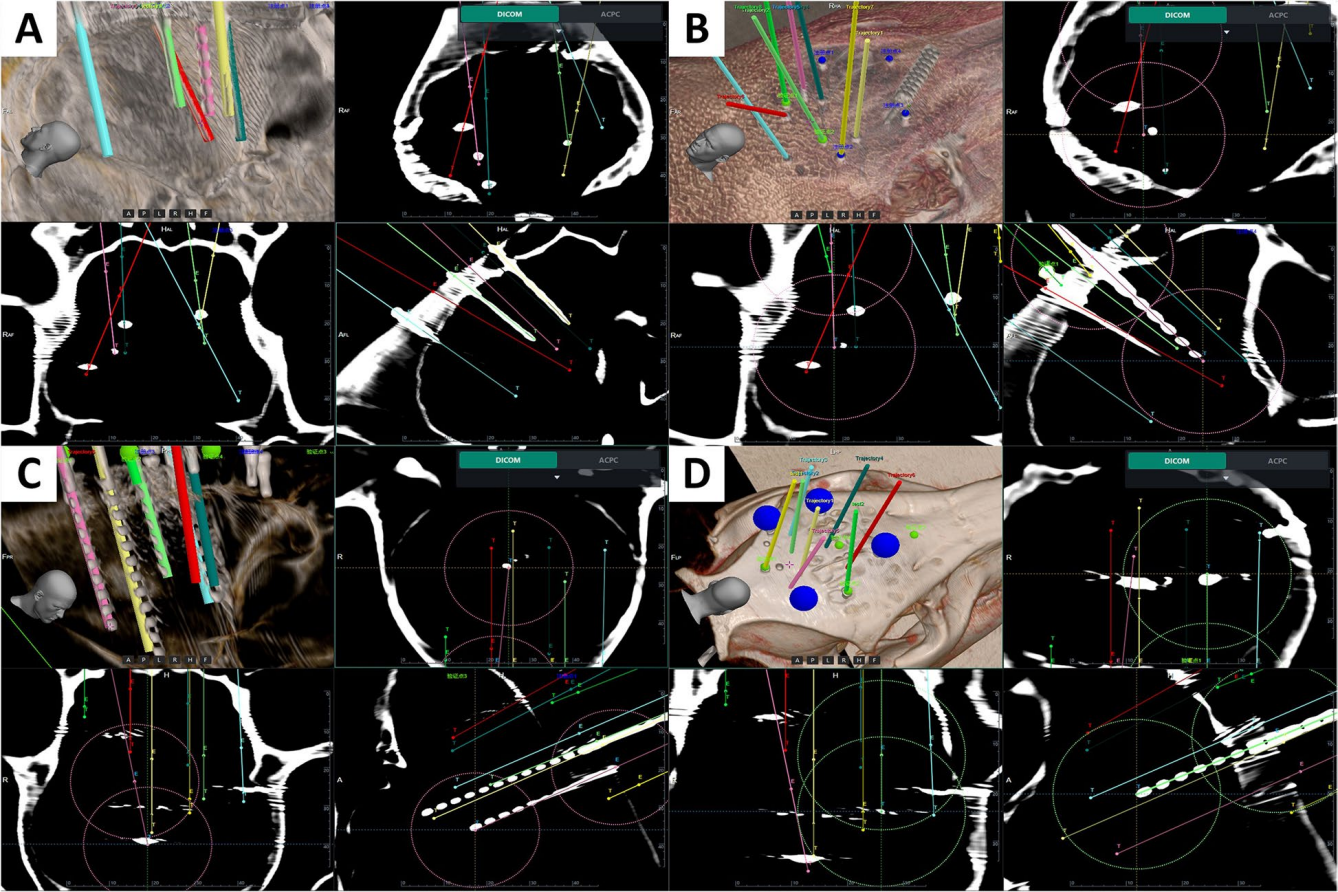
Planning MRI and postoperative CT verification image. A, B A case of postoperative analysis under CBR. C, D Another case of postoperative analysis under OTR
